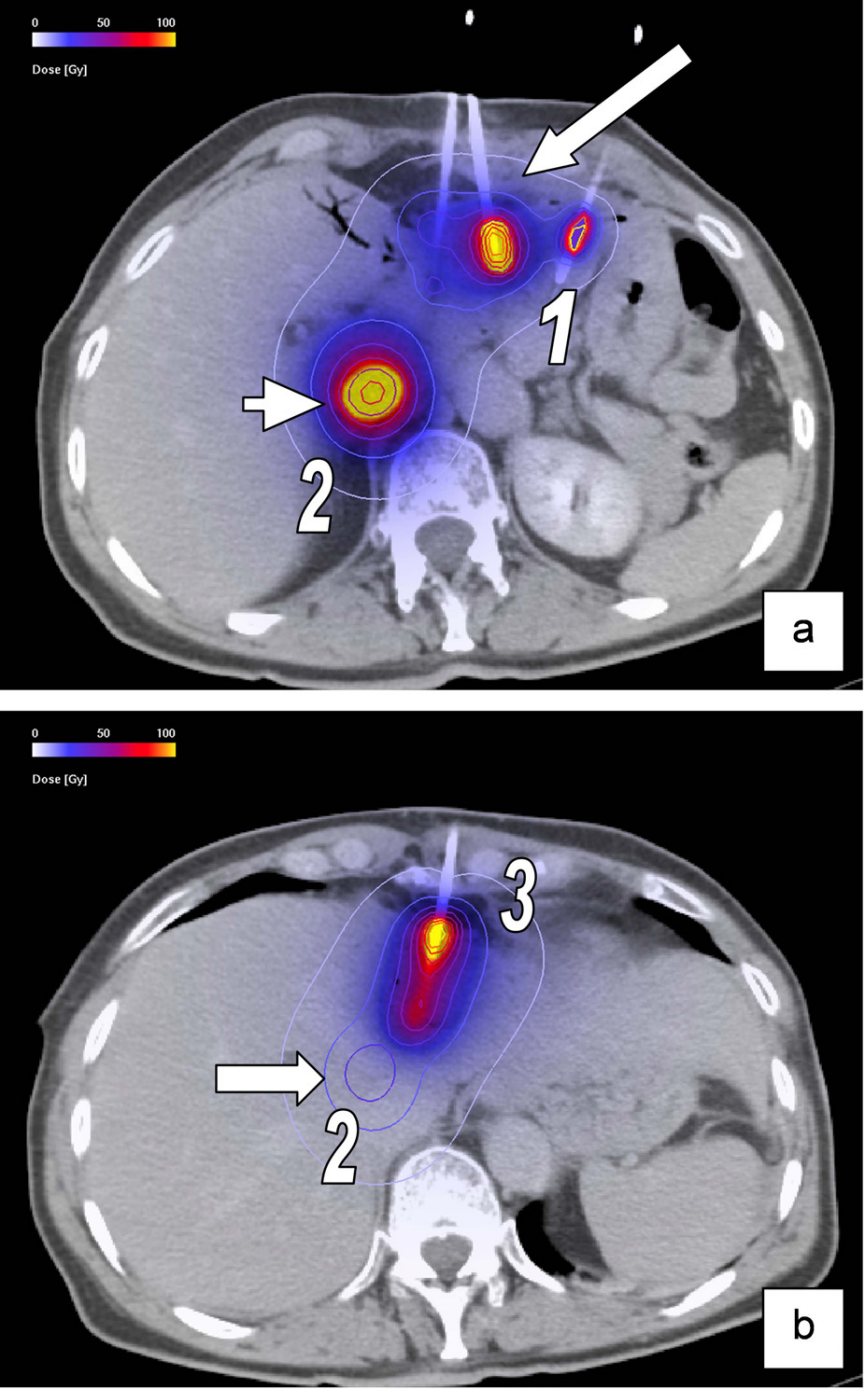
Image fusion of CT-data set with the accumulated 3-D dosimetry of three different irradiation sessions. (a) Contrast-enhanced CT after first (No.1) CT-guided positioning of brachytherapy catheters (long arrow) in metastases of a colorectal carcinoma. The short arrow shows the 3-D dosimetry of another lesion, irradiated in session No.2. (b) Contrast-enhanced CT after third intervention (No.3) in the same patient. The arrow shows the upper boundary of the 3-D dosimetry of the lesion irradiated in session No.2. Physical doses are shown in the colour map.
